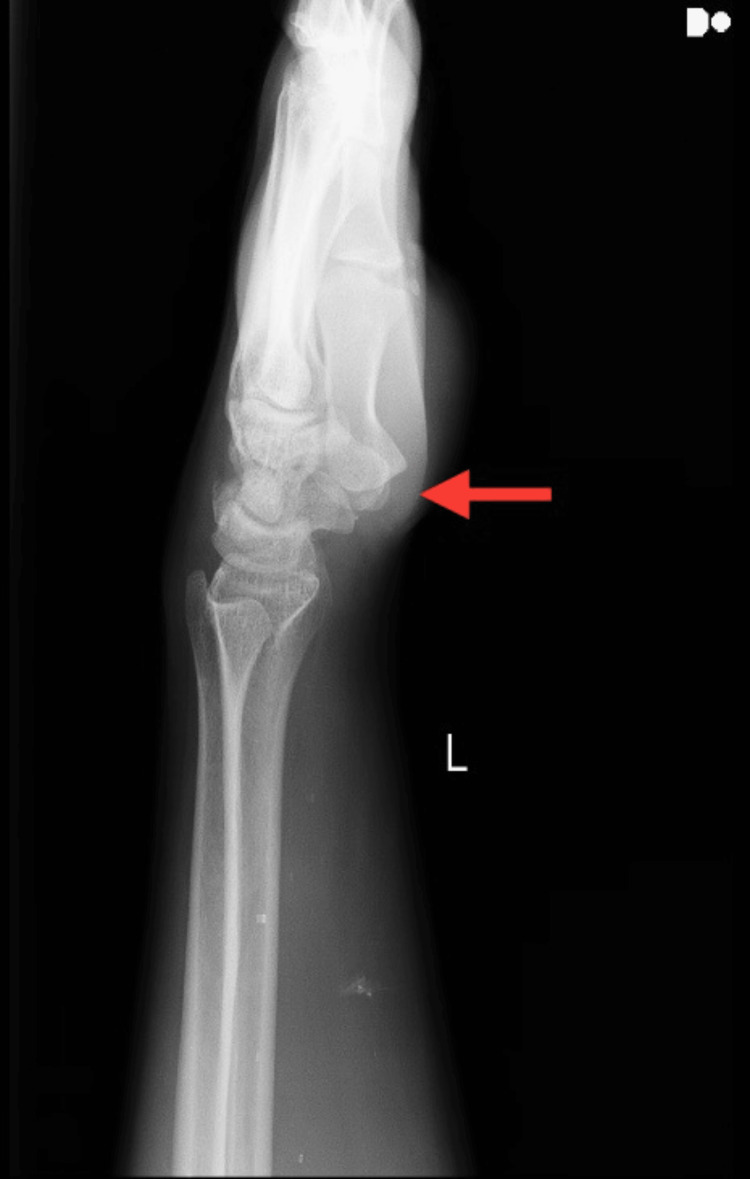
Radiograph of the left wrist in lateral view showing VISI VISI, volar intercalated segment instability
